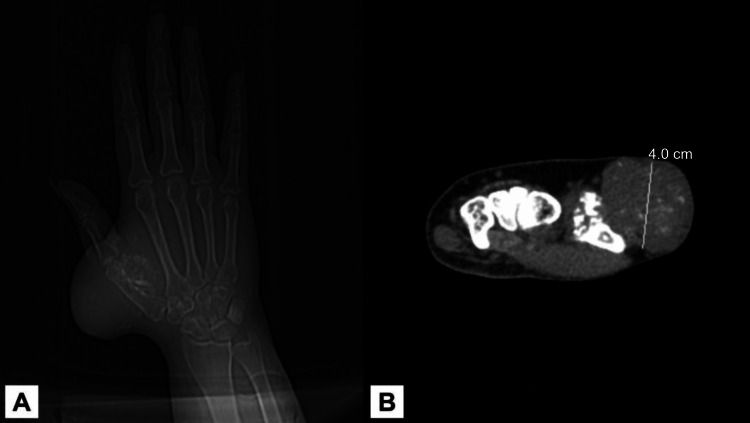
Radiological imaging findings (A) The radiograph of the left hand reveals the presence of a soft tissue mass with calcification; (B) the CT scan of the left hand indicates the existence of a lobulated mass that measures 4.0 cm in its largest anterior-posterior (AP) dimension. This mass extends into the webspace and is adjacent to the first metacarpal. The mass involves the first dorsal interosseous muscle and exhibits calcifications.
